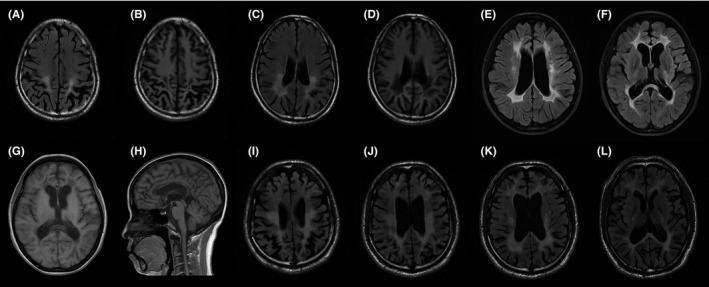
Brain MRI of patients with ALSP. A, B, C and D, MRI of proband 1 at his 43 showed the broadening and deepening of sulus with occipital prominence, and occipital periventricular white matter hyperintense on FLAIR and hypointense on T1‐weighted images. E, F, G and H, MRI of proband 2 at her 46 showed the white matter adjacent to the lateral ventricle hyperintense on FLAIR and hypointense on T1‐weighted images, and thinning of the corpus callosum. I, J, K and L, MRI of proband 3 at his 38 showed widespread white matter hyperintense and obvious cortical atrophy on FLAIR
